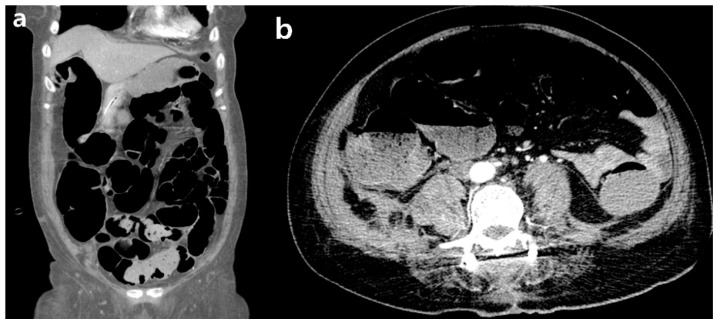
Abdominal CT angiography showed a severe small-bowel paralytic ileus. (a) Coronal; (b) axial cut.
